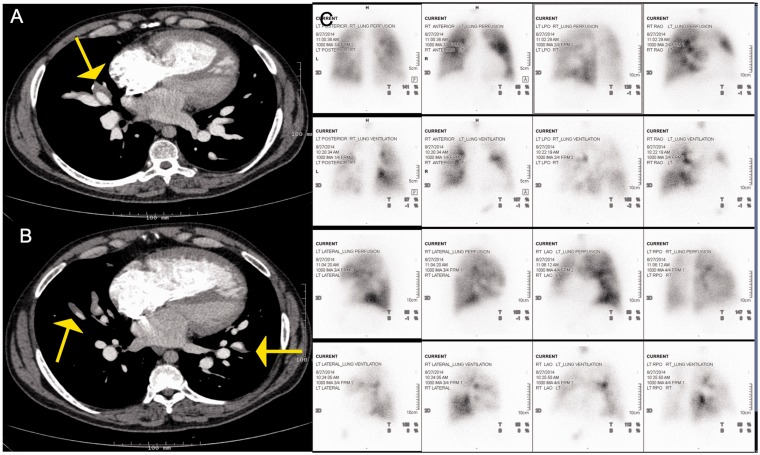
Panel A and B: Contrast enhanced CT images in a 50-year-old patient
